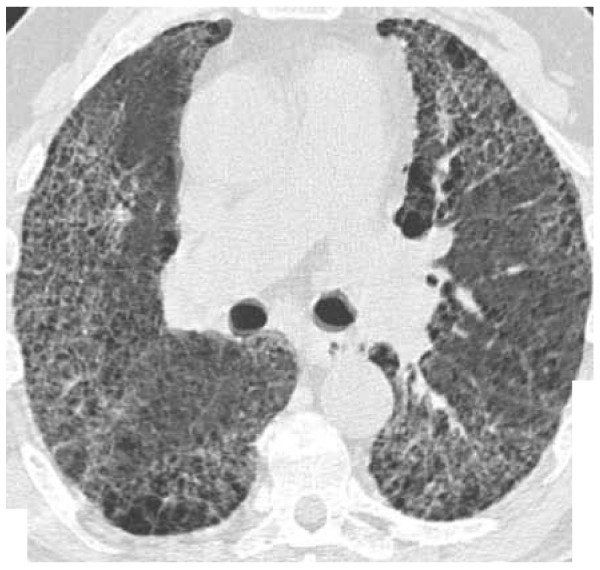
Computed tomography scan of the thorax showing extensive honeycombing in both lungs with widespread ground glass opacification and patchy asymmetrical areas of normal lung tissue.
